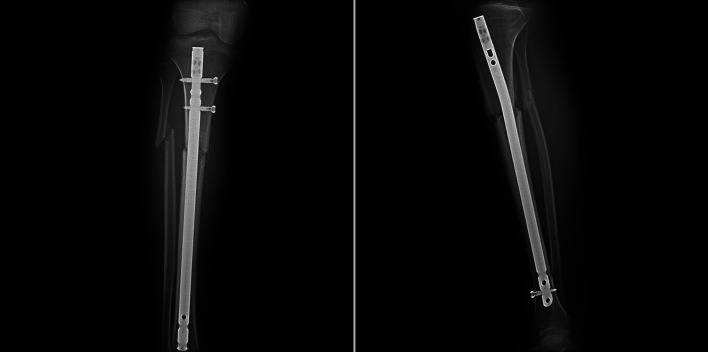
X-ray images of an exemplary case of fractures distal to the isthmus.
